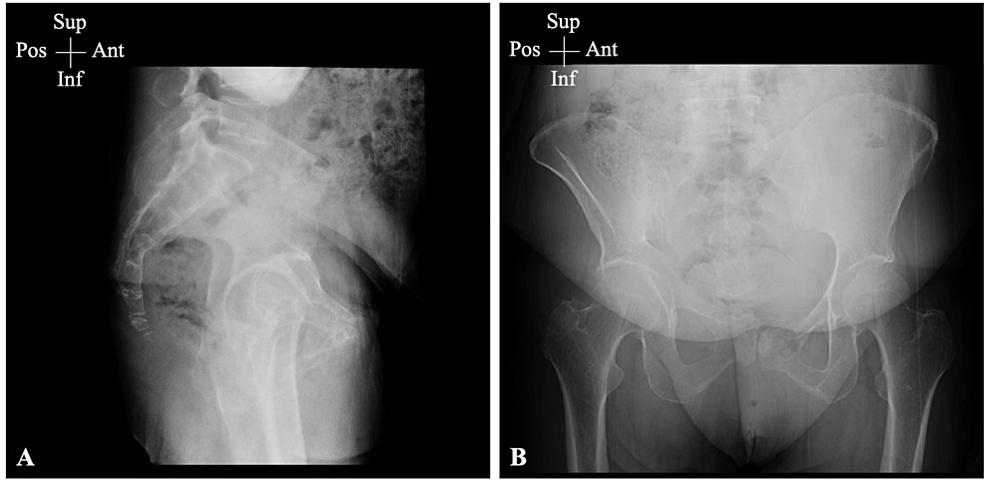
Plain pelvic X-ray Lateral (A) and posteroanterior (B) views of the pelvic plain X-ray. Although the ingested FB was of bone contrast, a plain X-ray failed to provide its shape and dimensions FB: foreign body; Sup: superior; Inf: inferior; Ant: anterior; Pos: posterior
